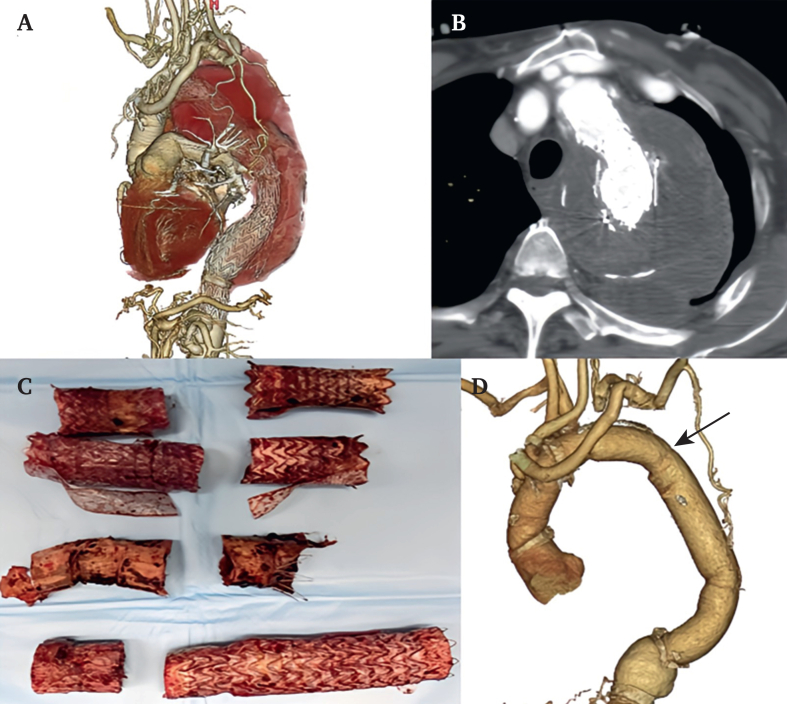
Pre- and post-operative examinations in cases of type V endoleak. (A, B) Pre-operative computed tomography (CT) shows an enlarged aortic aneurysm up to 10 cm despite multiple stent graft procedures. (C) Extracted stent grafts including cuffs in this case. (D) CT demonstrates the reconstructed aorta with prosthetic graft following complete extraction of the stent graft (arrow). Anastomosis of a prior elephant trunk to a new prosthetic graft.
